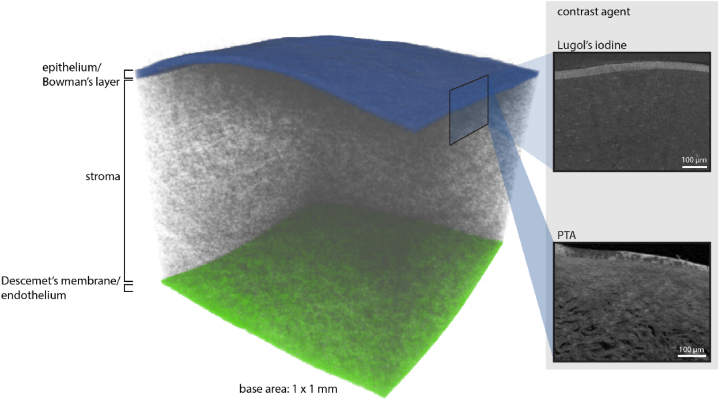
3D structure of the human cornea visualized by Nano-CT. Different contrast-enhancement agents for better visualization of the cornea structure.
